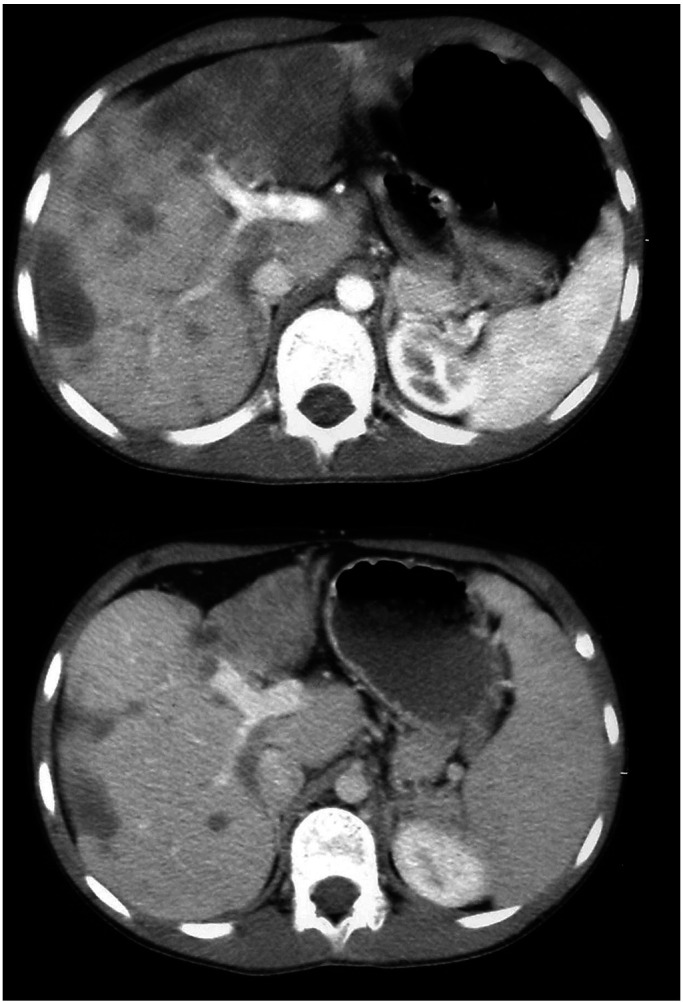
Hepatic epitheloid haemangio-endothelioma in a 10-year-old child. CT scan aspect before and after chemotherapy (carboplatin, etoposide), showing some response to chemotherapy that was associated with resolution of clinical symptoms.
